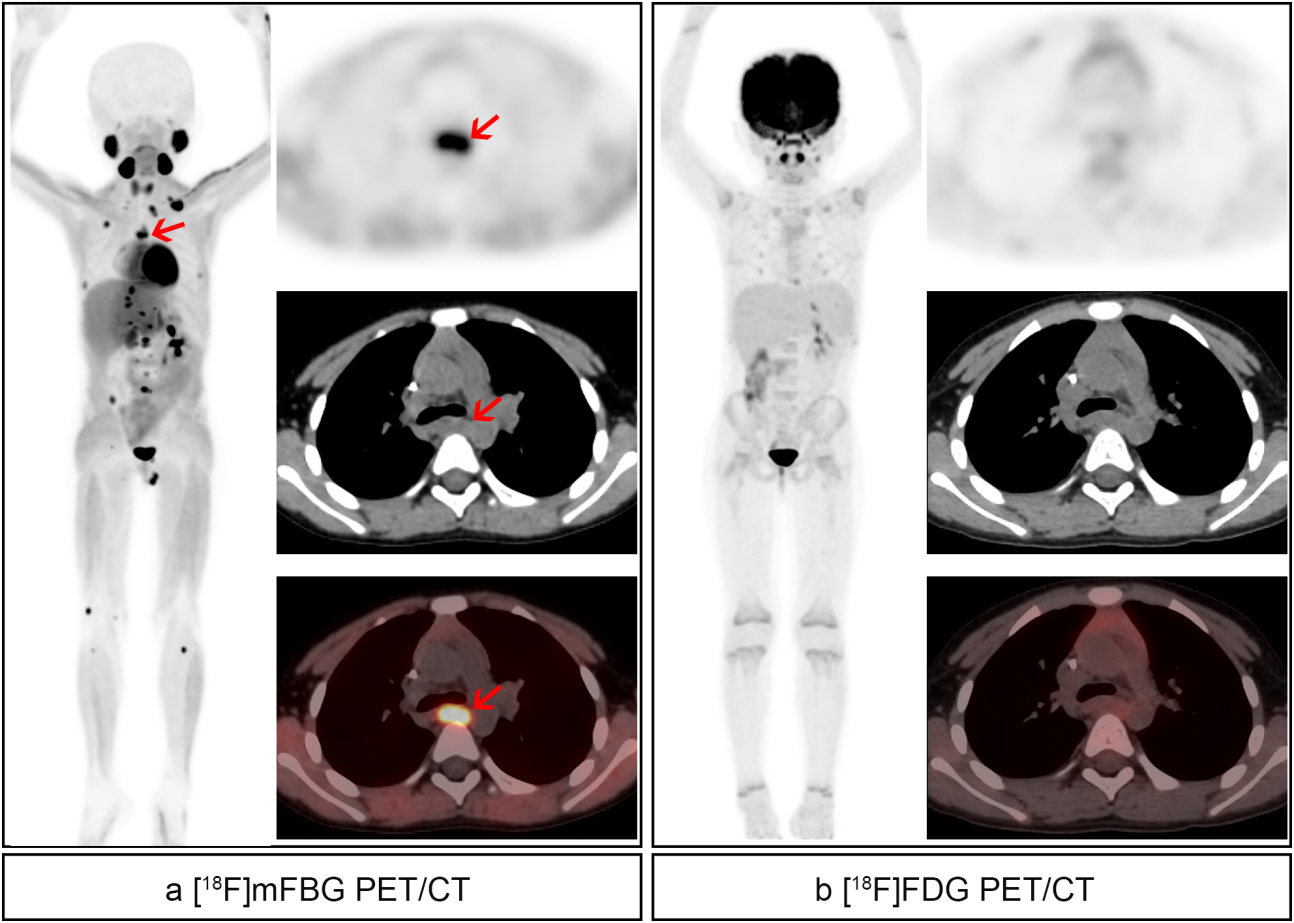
Therapy response evaluation of a 4-year-old boy suffered high-risk right retroperitoneal neuroblastoma underwent resection of lesions followed by 3 cycles of chemotherapy. (a) [^18^F]mFBG images. Pathological uptake was found in extensive lymph nodes and bone/bone marrow (red arrows). (b) [^18^F]FDG images. No residual activity was found.
